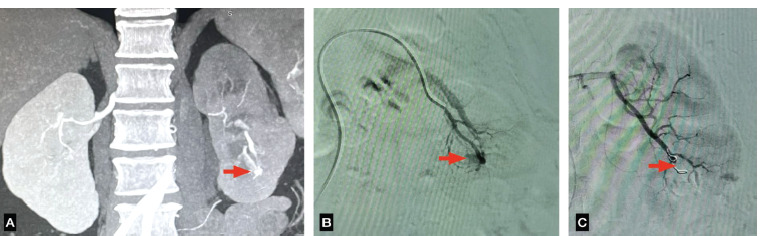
(A) CT Angiography showing an arteriovenous fistula in the lower polar left renal artery with an associated pseudo aneurysm. (B) Diagnostic angiogram showing an arteriovenous fistula and a pseudo aneurysm in the left lower polar artery. (C) Post embolisation showing two coils in situ leading to occlusion of both the pseudo aneurysm an AV fistula.
